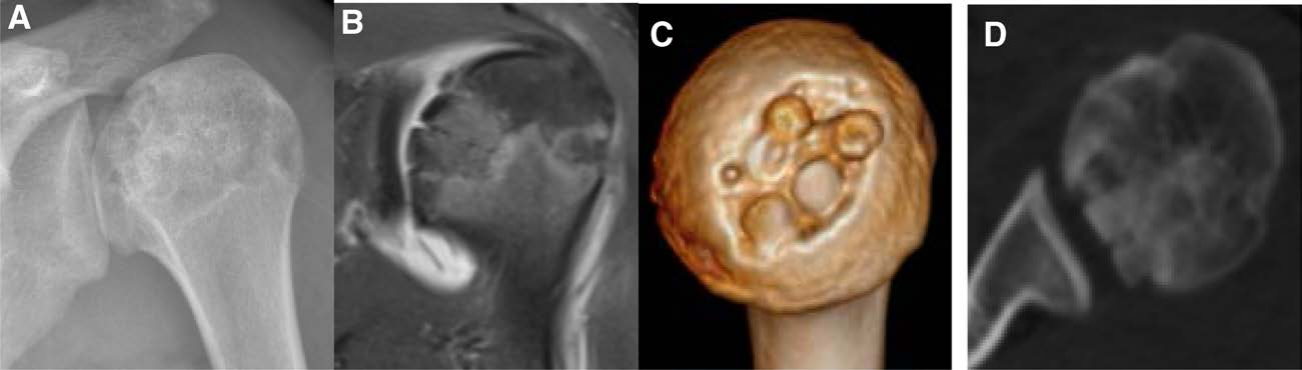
The postoperative X-ray, MRI T2-weighted image, and CT + 3D CT scan showed that the shape of the left humeral head was significantly improved compared with preoperative, the necrosis was removed, autologous cartilage was implanted, and the articular surface was repaired. CT = computed tomography, MRI = Magnetic resonance image.
